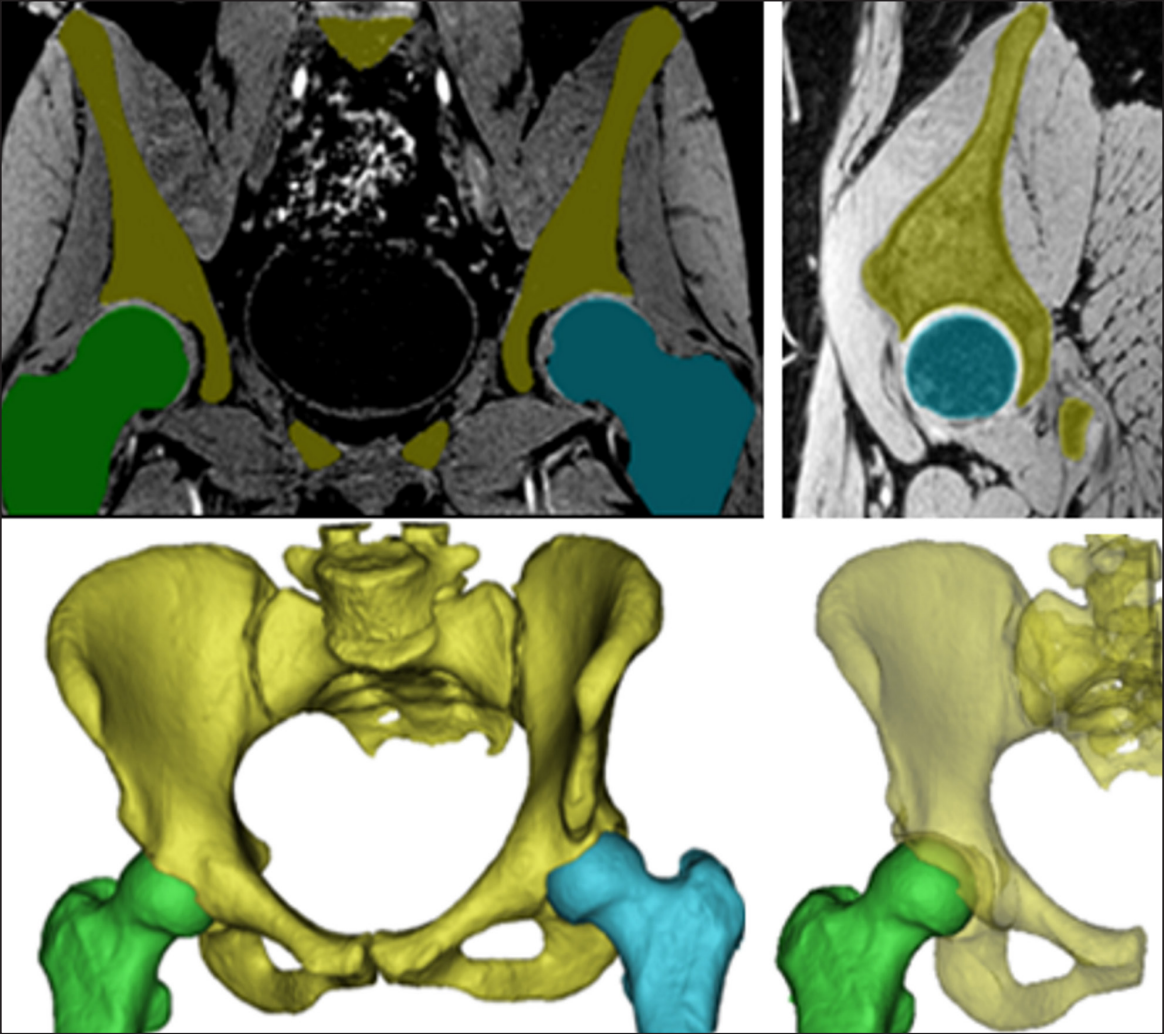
The 3D nature of the MRI-based synthetic CT reconstruction facilitates multiplanar reconstructions, 3D renderings and automated segmentation (Courtesy of MRIguidance, University Medical Center Utrecht, The Netherlands; boneMRI v1.4. MRIguidance BV, Utrecht, The Netherlands).
